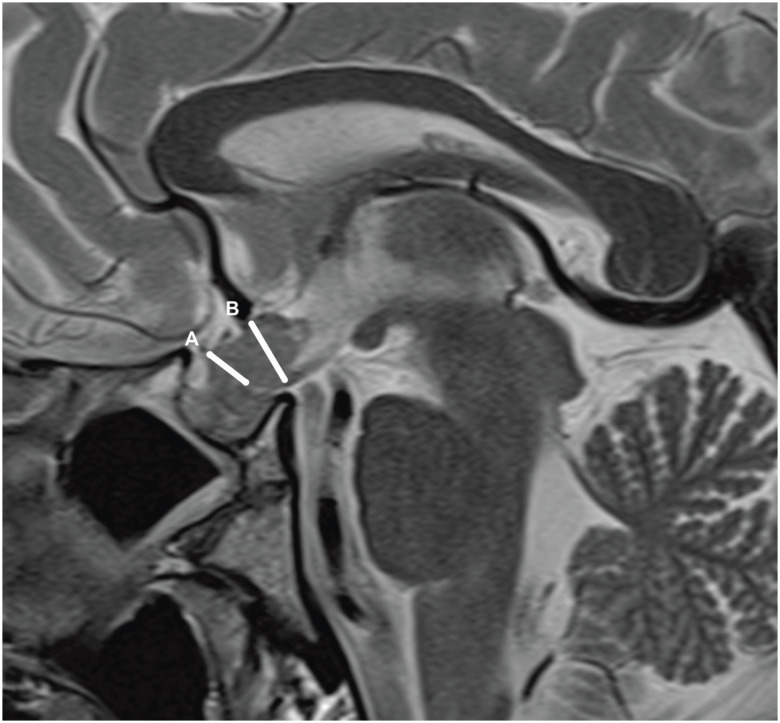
Magnetic resonance imaging of a patient with pituitary stalk thickening at the (A) pituitary insertion and (B) optic chiasm.
